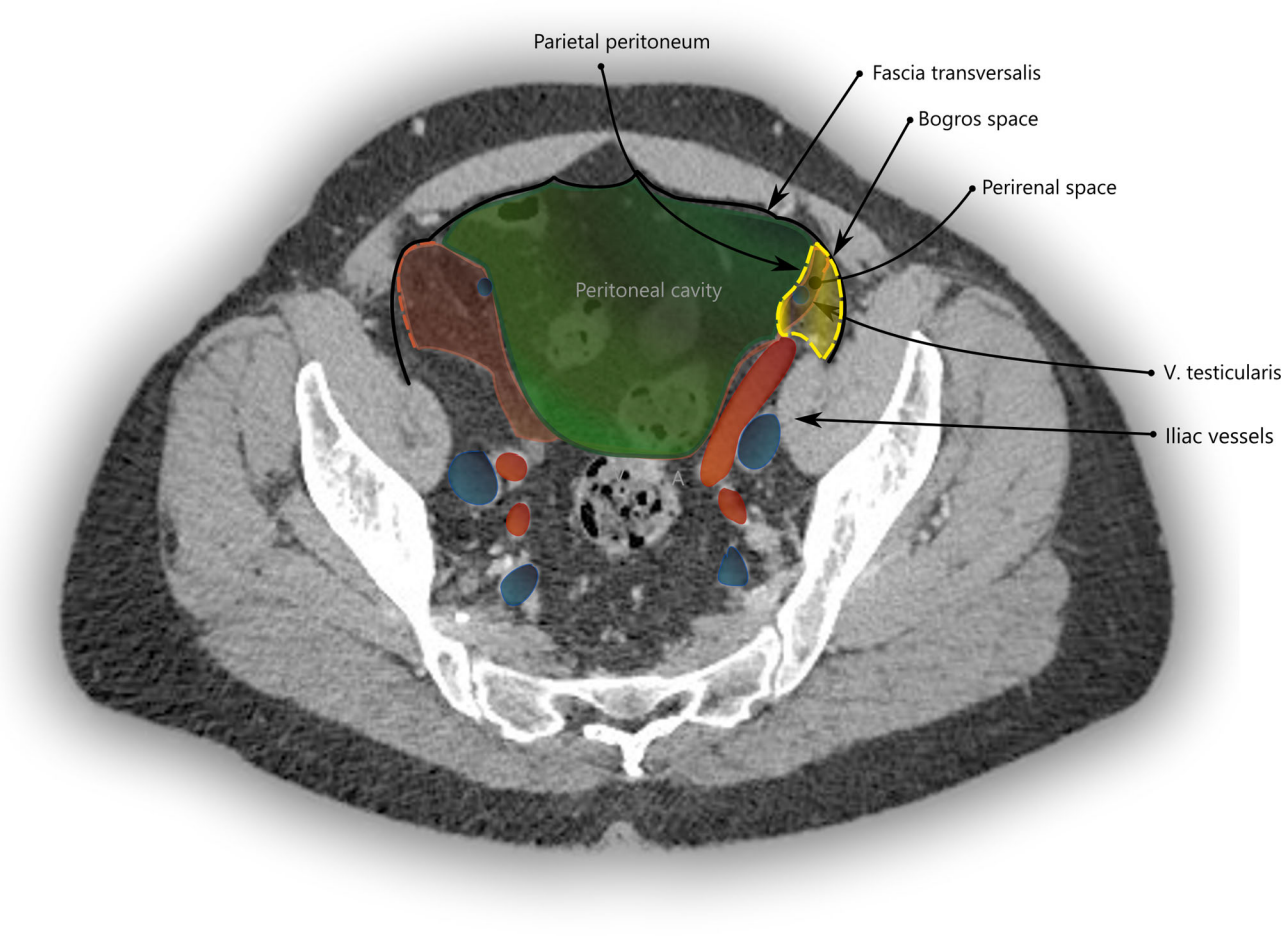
CT imaging with a transverse section through the pelvis at the apex of the urinary bladder. The space of Bogros is marked in yellow and limited by the TF (black) laterally and the parietal peritoneum (green) medially. It contains the extension of the perirenal space (red) into the pelvis.
